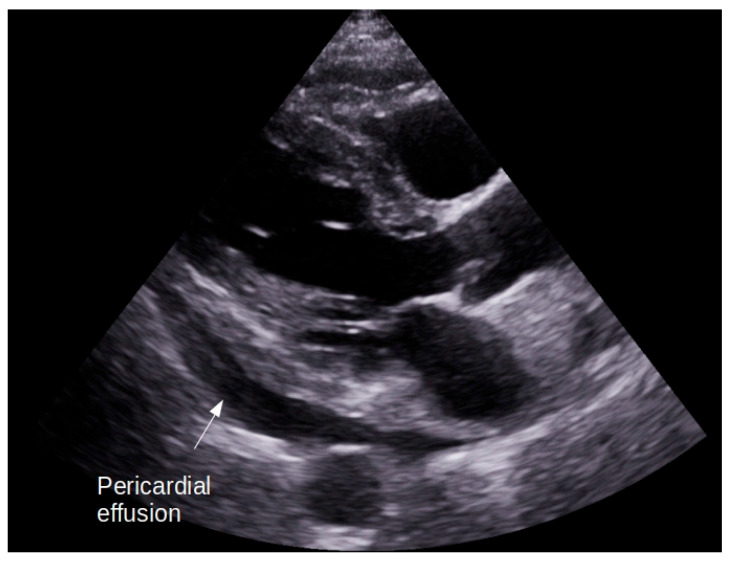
Parasternal long-axis view with pericardial effusion (image courtesy of E. Polyzogopoulou).
